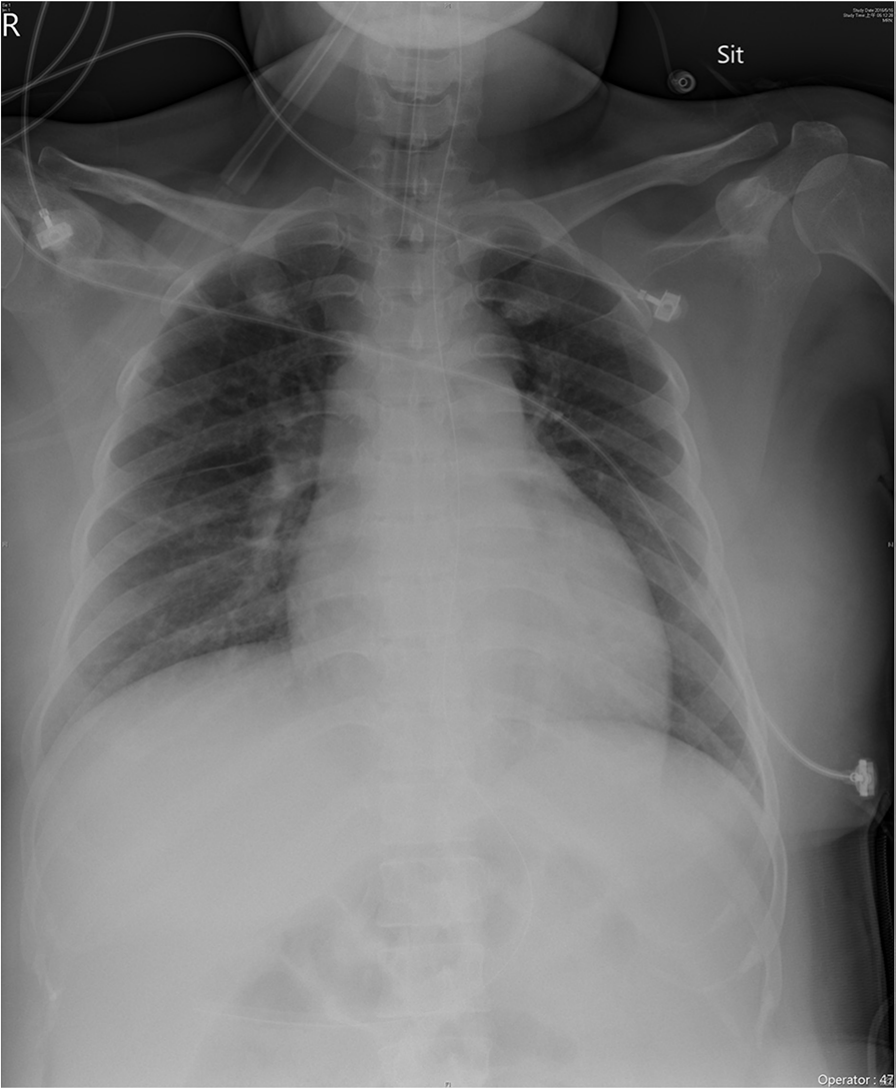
Chest radiograph revealed mild bilateral infiltration
